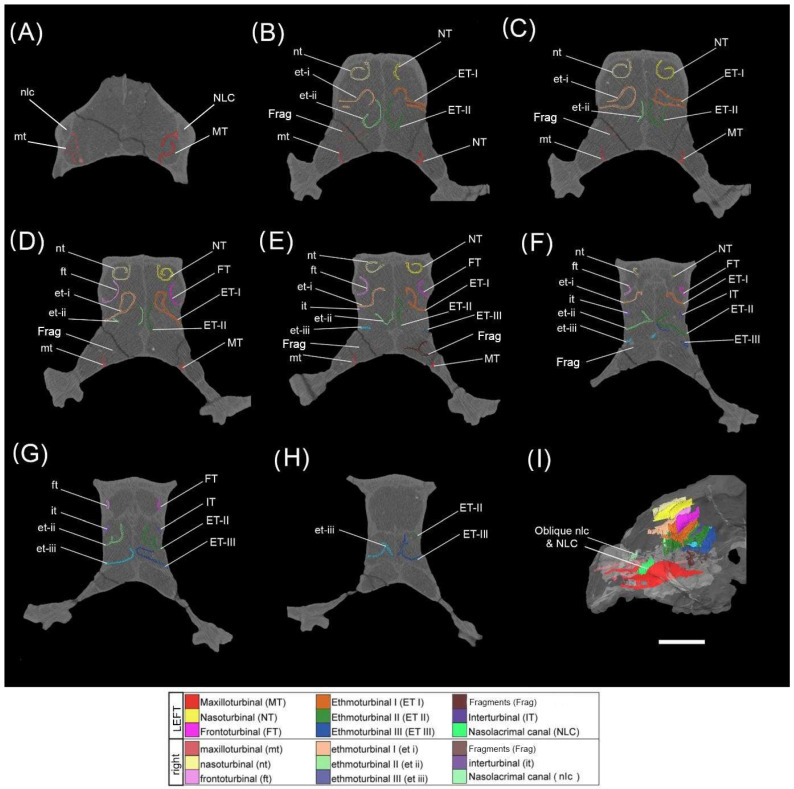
CT scans through the nasal fossa of the holotype of *Mioeuoticus shipmani* (KNM-RU 2052). (A–H) Slices along the coronal plane showing preservation of left and right nasal fossae: slice number 97 (A), 137 (B), 139 (C), 146 (D), 156 (E), 173 (F), 179 (G), and 190 (H) of 449 total slices. (I) Digitally bisected volume renderings of the cranium showing posterior nasal fossa anatomy in *Mioeuoticus*. Frag = unidentified floating turbinal fragment. Scale bar is 10 mm.
